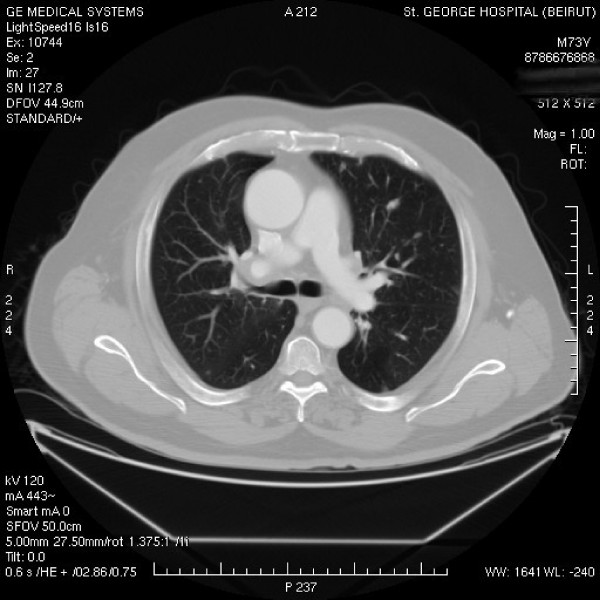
CT-scan taken at the level of the carina. Remarkable decrease in size and number of the nodular metastases as well as of the hilar lymph nodes.
